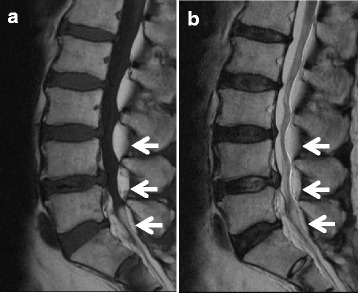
Lumbar sagittal magnetic resonance imaging. a T1-weighted sagittal image, b T2-weighted sagittal image. Arrows indicate lumbar epidural fat
